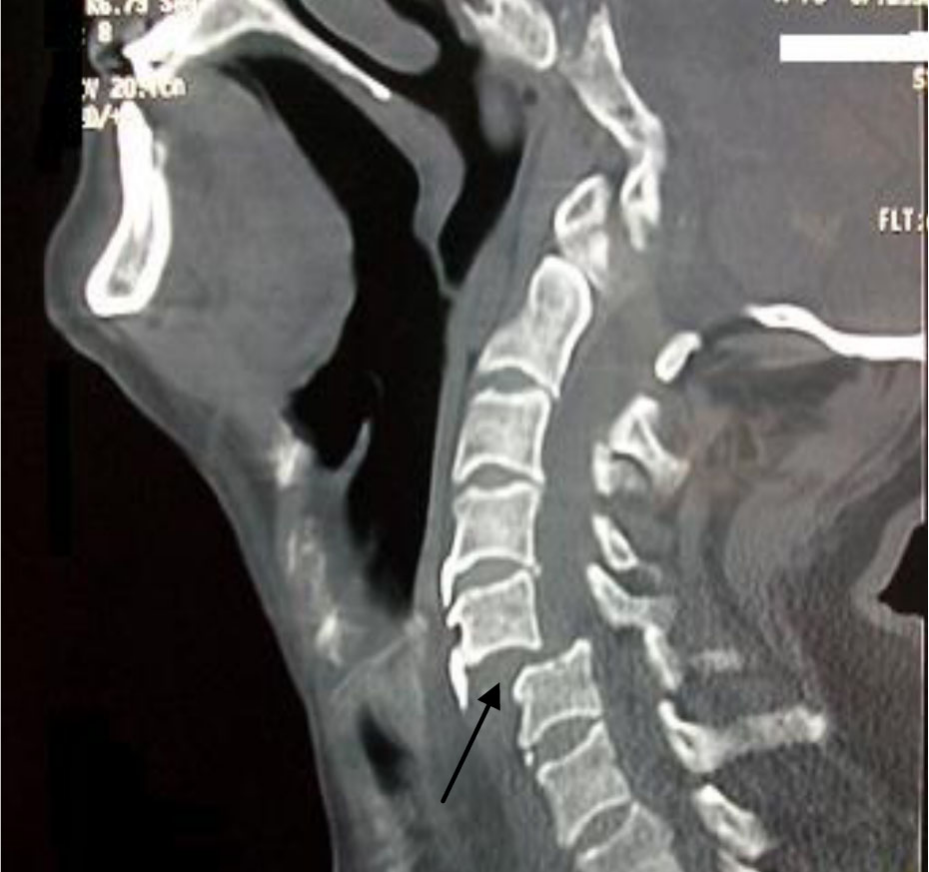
CT scan of cervical spine revealing partial anterior sublaxation (C5 over C6 cervical vertebrae).
